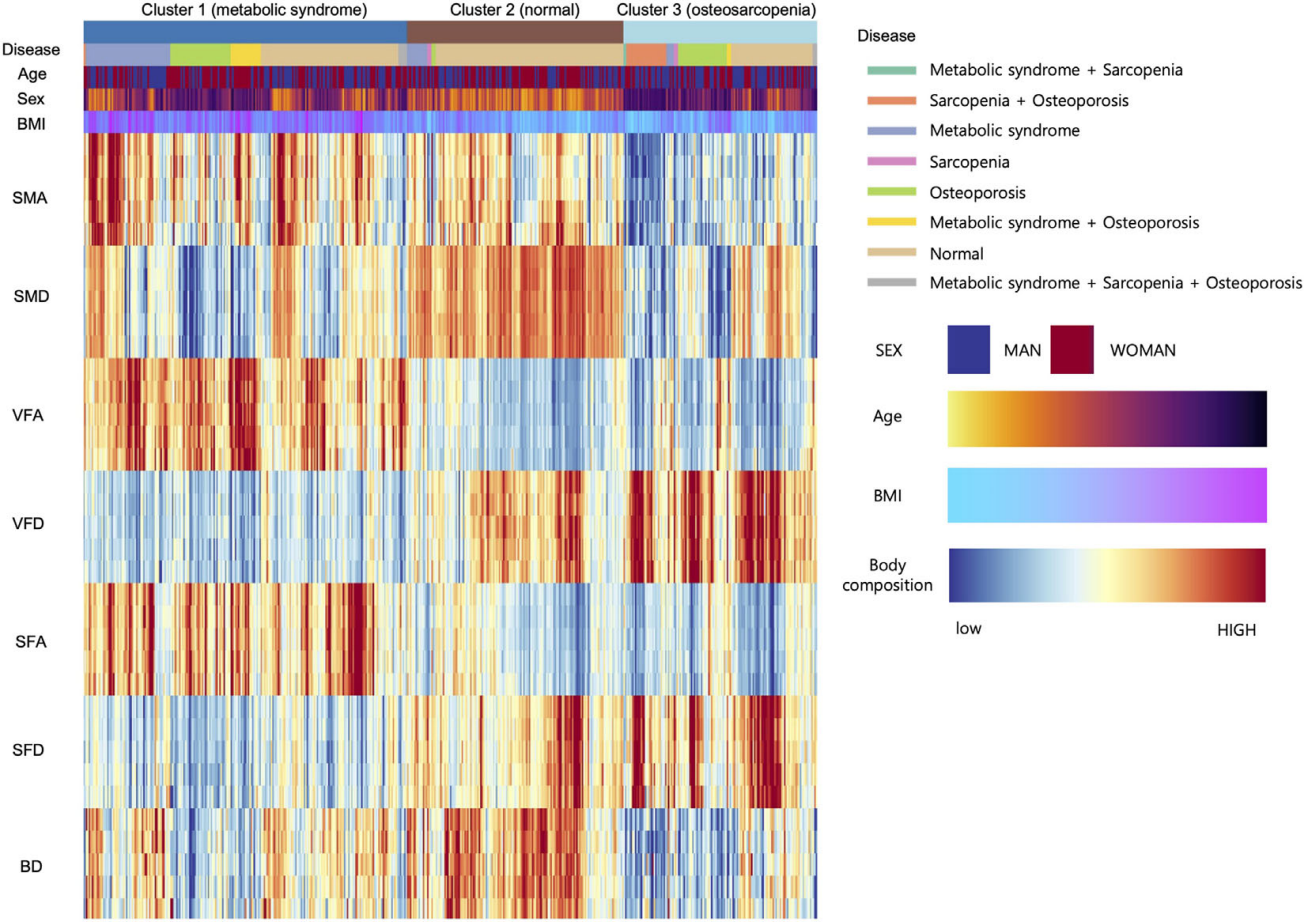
Computed tomography body composition‐based metabolic phenotype clusters obtained using unsupervised hierarchical clustering of the derivation cohort. BD, bone density; BMI, body mass index; SFA, subcutaneous fat area; SFD, subcutaneous fat density; SMA, skeletal muscle area; SMD, skeletal muscle density; VFA, visceral fat area; VFD, visceral fat density.
